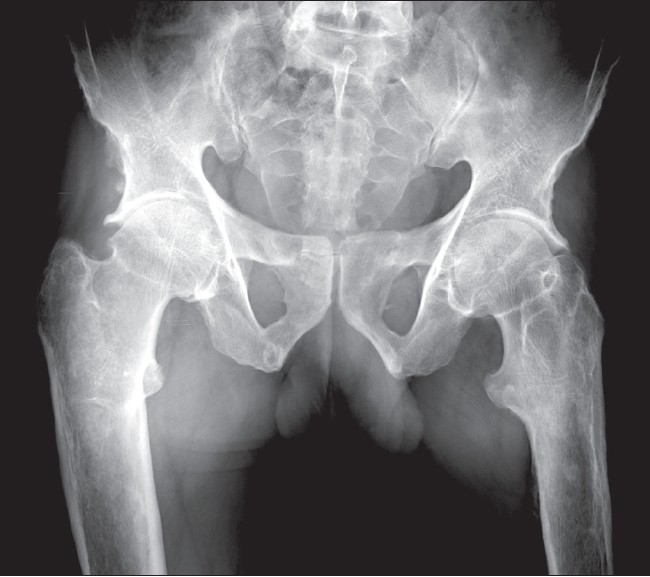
Anteroposterior radiograph of the pelvis (including both hips) shows symmetric and shaggy periosteal proliferation in the lower part of the iliac bones. There is widening of the femoral metaphyses with reduction of hip joint space on both sides, without any abnormality of the articular surface
